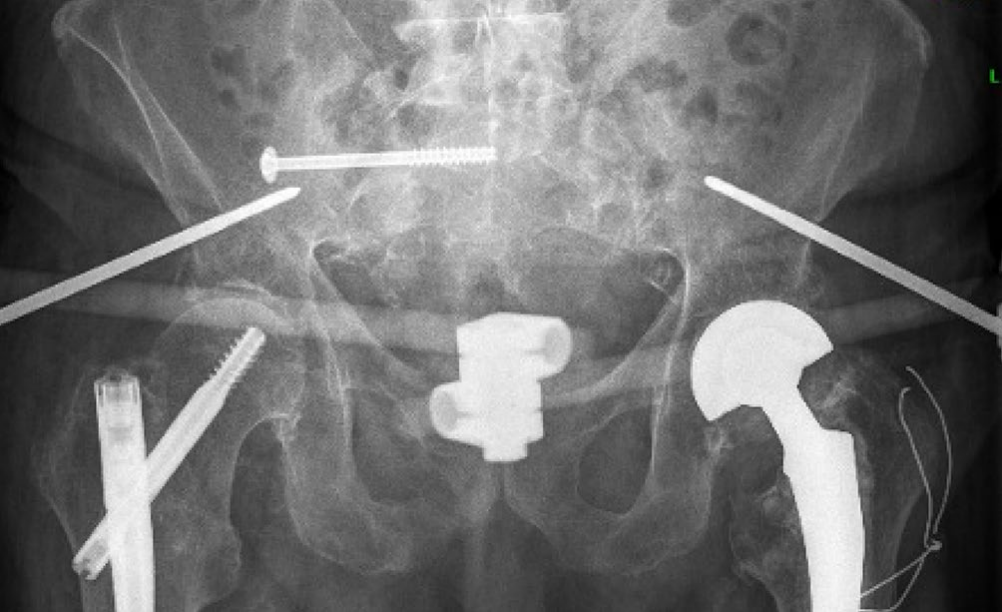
Showing an X-ray postoperatively after percutaneous S1 Screw fixation and application of anterior supraacetabular external fixator.
